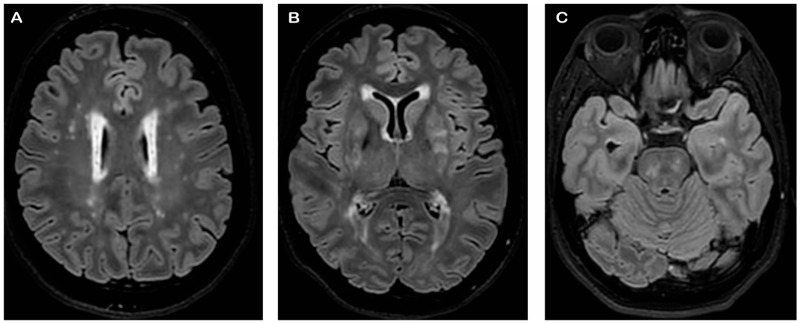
Patient 1’s FLAIR MRI axial plane showing multiple punctate hyperintensities in the deep white matter and confluent lesions in the periventricular white matter (A), minimal involvement of external capsules (B) and anterior temporal poles and more severe extension to the pons (C).
